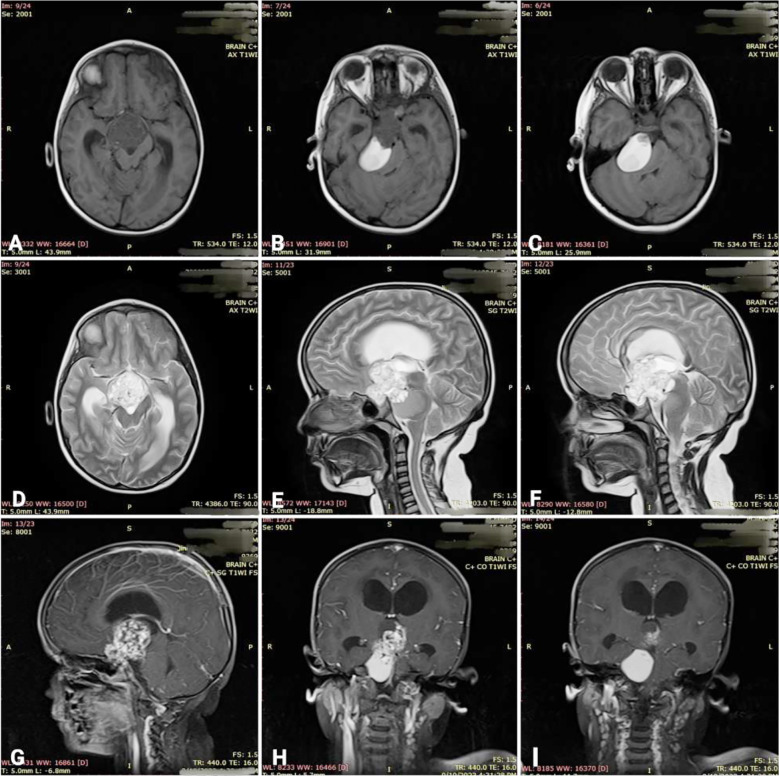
A, B & C: T1-weighted axial images showing a hypointense (solid) lesion in the sellar region and a hyperintense (cystic) infratentorial lesion. D: T2-weighted axial image showing hyperintense lesion. E & F: T2-weighted sagittal images showing the same lesion. G: T1-weighted contrast axial image showing heterogeneously enhancing lesion. H & F: T1-weighted contrast coronal images showing a heterogeneous lesion in the sellar/suprasellar region and a hyperintense infratentorial lesion.
